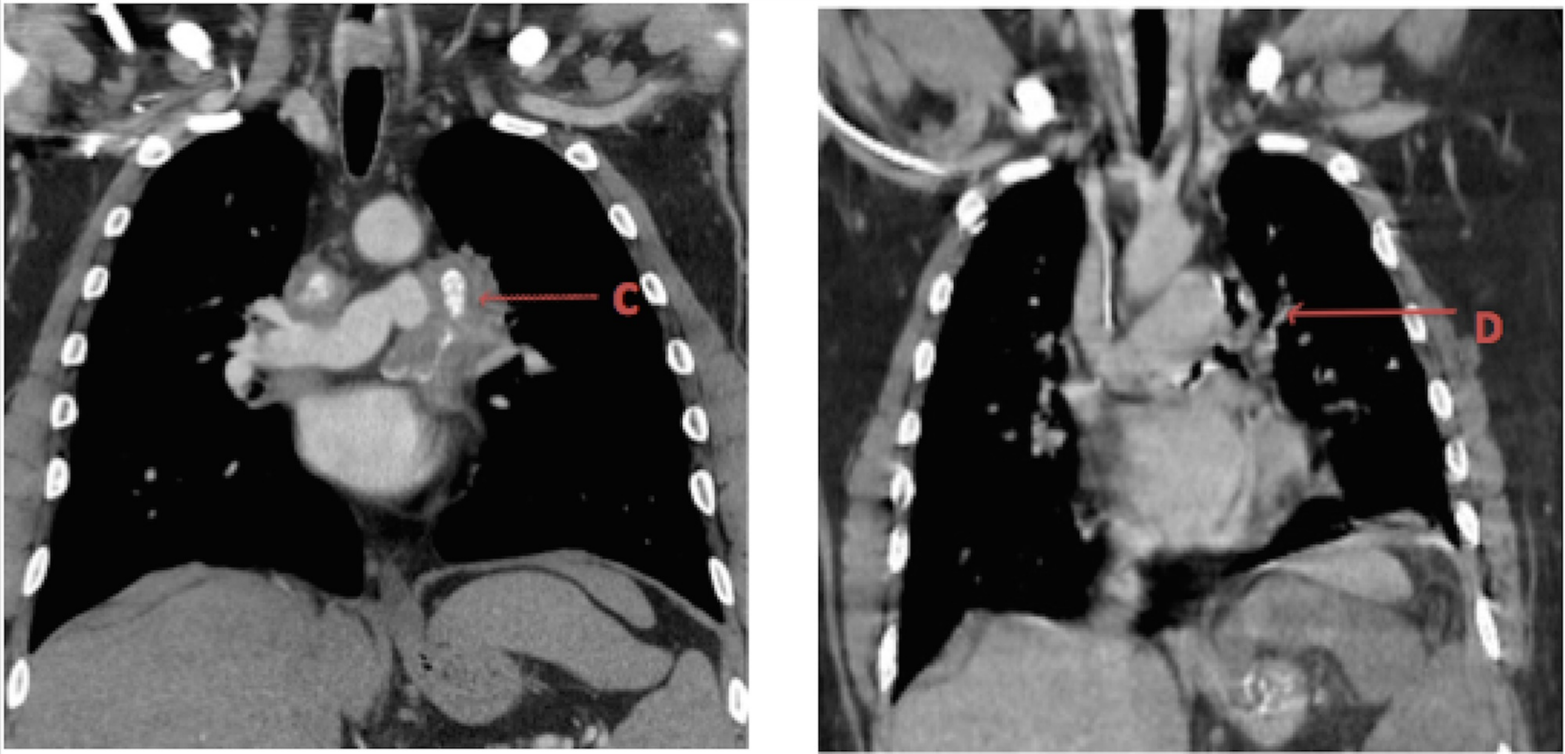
CT images comparing previous (left) and current (right) imaging with lymph node eroding into the left bronchus and upper pulmonary vein with new pneumomediastinum. C: Fibrosing mediastinitis seen in the CT chest two years ago. D: Erosion of lymph node into the left bronchus and the left upper pulmonary vein
